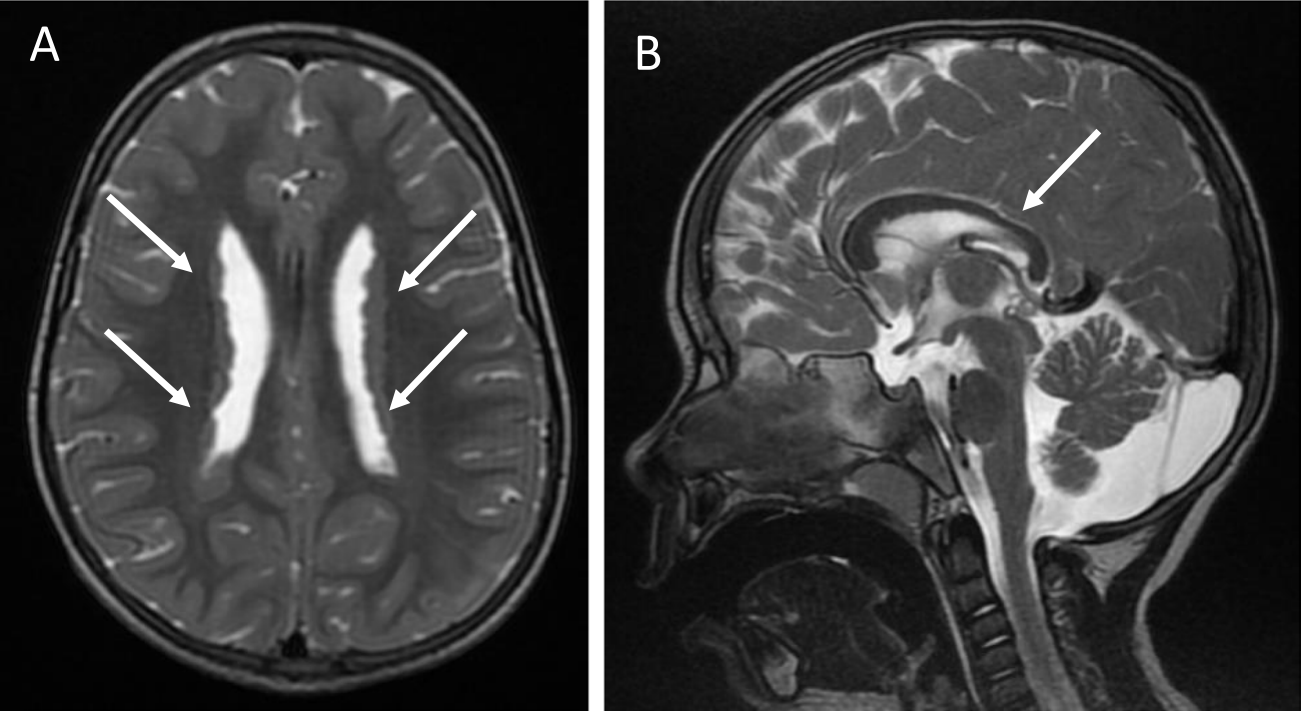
Brain imaging findings from MRI performed at age 4 (Twin B). A Axial T2-weighted images: Bilateral symmetric subependymal heterotopia is noted (arrows). The ventricles are normal in size, with no signs of hydrocephalus. B Midline sagittal T2-weighted image: The corpus callosum is slightly short, with mild thinning observed in the posterior portion, including the posterior body, isthmus, and splenium (arrow). In the posterior fossa, the vermis is relatively small (cranio-caudal dimensions), displaying features consistent with inferior vermian hypoplasia. Additionally, prominent CSF spaces and an enlarged cisterna magna are present in the posterior fossa
